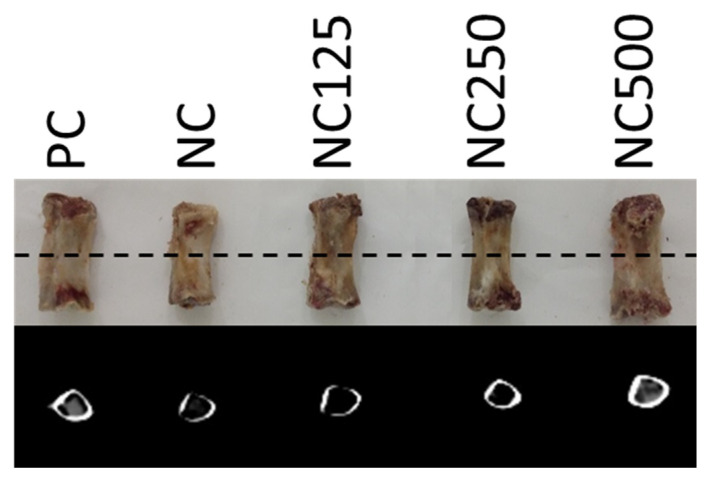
Example of disposition of the bones (one of each treatment) for the computed tomography (CT) acquisition and axial image obtained of the centre of the bones (discontinuous line).
